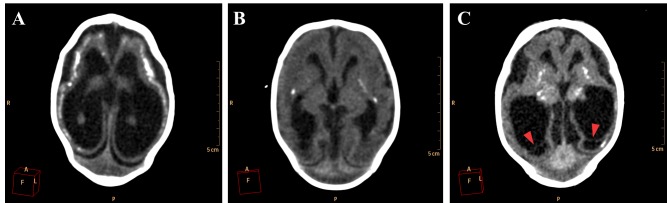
Figure 4. Axial non-contrast brain CT with three different patterns of parenchymal atrophy and compensatory ventricular dilation. Ventricular adherences are suggested by the presence of thin septations with soft tissue density observed in the occipital horns of lateral ventricle (red arrowheads in C).
